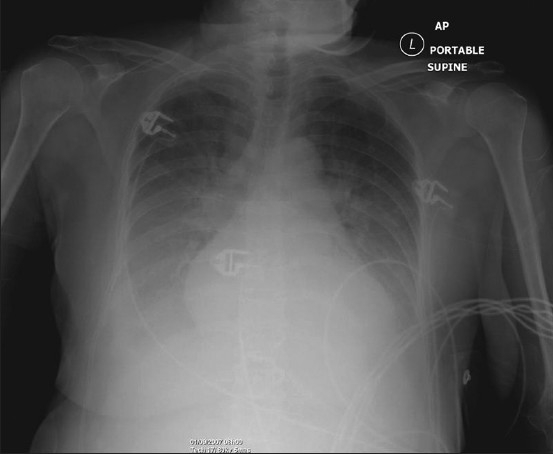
Frontal chest radiograph showing features of alveolar pulmonary edema. The findings include opacification of both lungs with increasing density towards the lung bases due to a combination of air space shadowing and pleural effusions, cardiomegaly, upper lobe blood diversion (unreliable on supine AP radiograph) and an air bronchogram in the right upper zone
